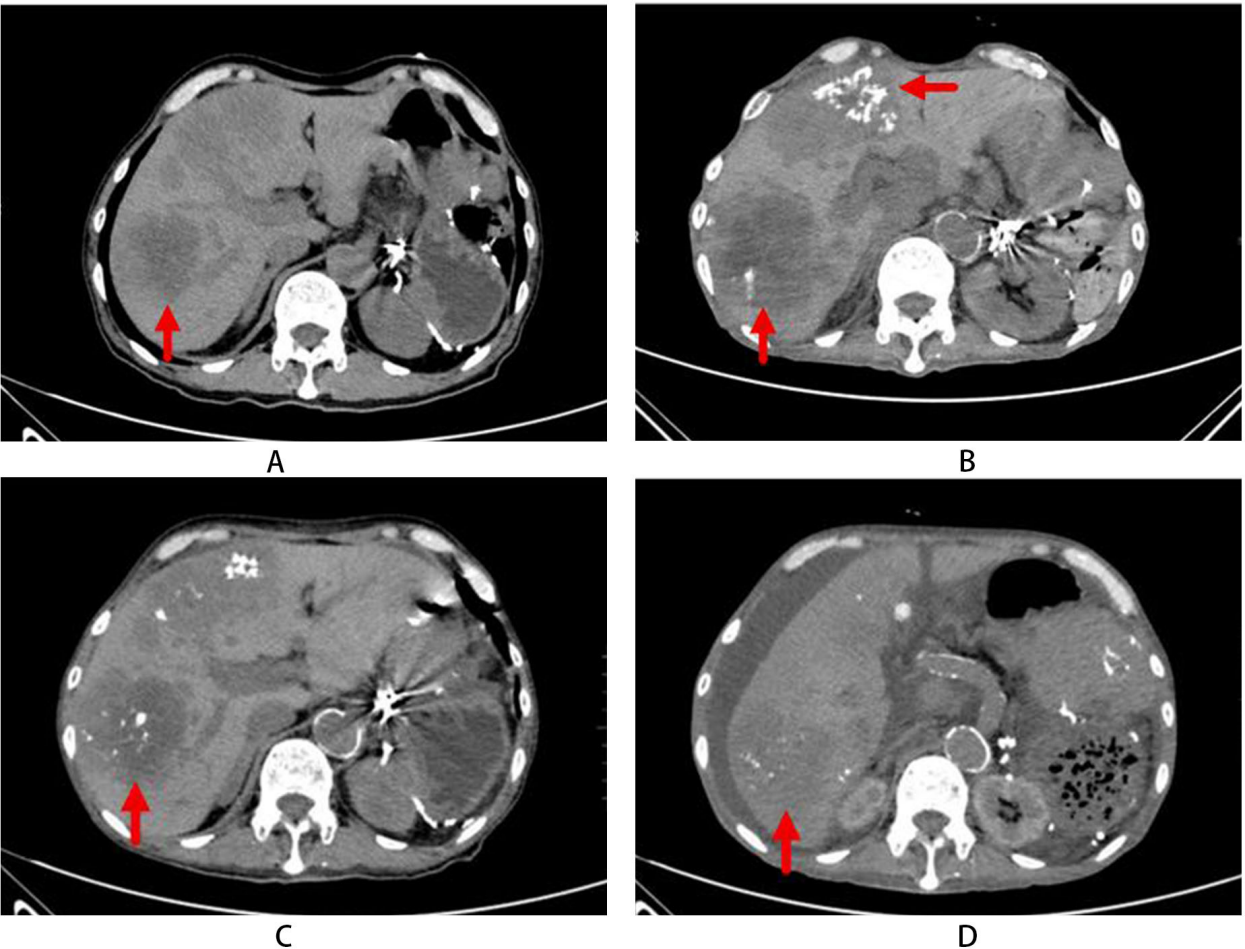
(A) (Mar 2023): The liver appears enlarged with preserved overall morphology. Multiple patchy and mass-like hypodense lesions are observed, consistent with diffuse hepatic metastases. (B) (Mar 2024): Follow-up CT post-TAE of hepatic metastases shows an increase in the size of target lesions compared with the prior study. (C) (Jul 2024): Subsequent CT post-TAE demonstrates that the hepatic metastatic lesions remain largely stable in size and appearance. (D) (May 2025): Further follow-up CT post-TAE indicates overall stability of the hepatic metastases, with no significant interval change.
